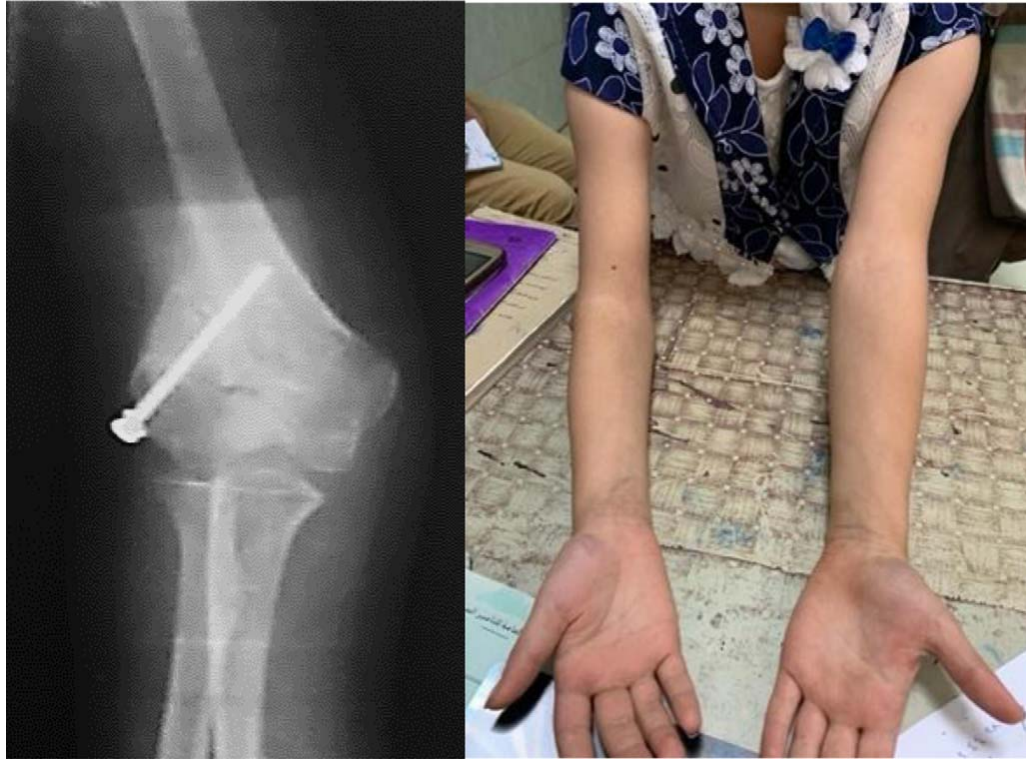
Postoperative X-ray and 7-month postoperative clinical photo of case no. (3) (right elbow).
